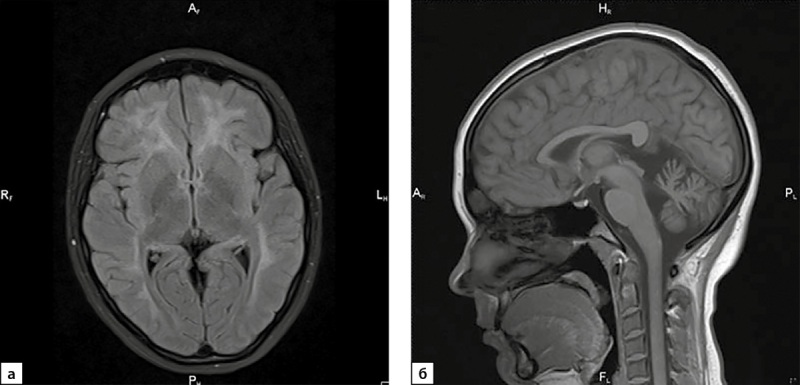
Рисунок 1. МРТ головного мозга пациентки с POLR3-ассоциированной лейкодистрофией (а — axial, Т2 FLAIR; б — saggital, T1).
